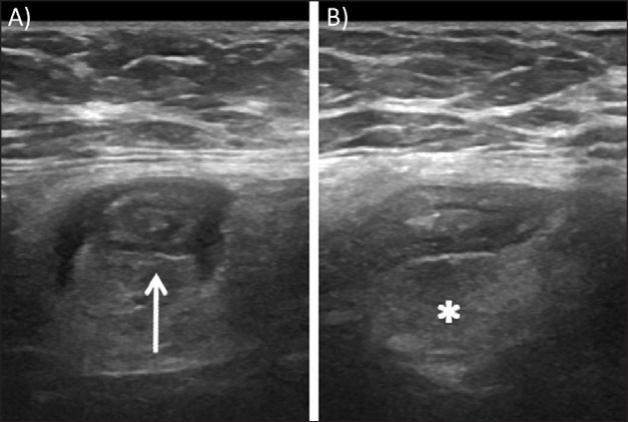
Grey-scale ultrasound. Axial (A) and sagittal views of the appendix within an inguinal hernia sac (B).
